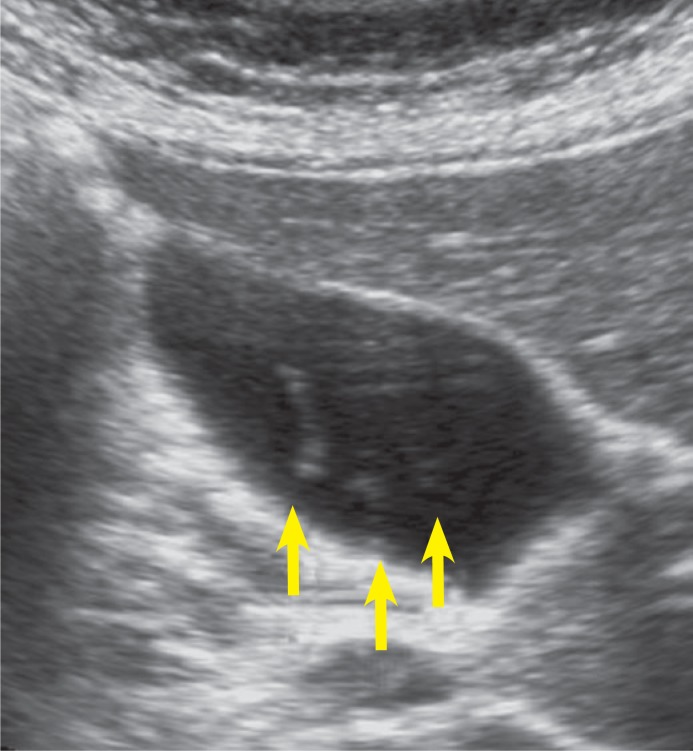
Transverse sonogram shows floating echoes (*arrows*) with no acoustic shadowing in the gallbladder of patient with fascioliasis.
